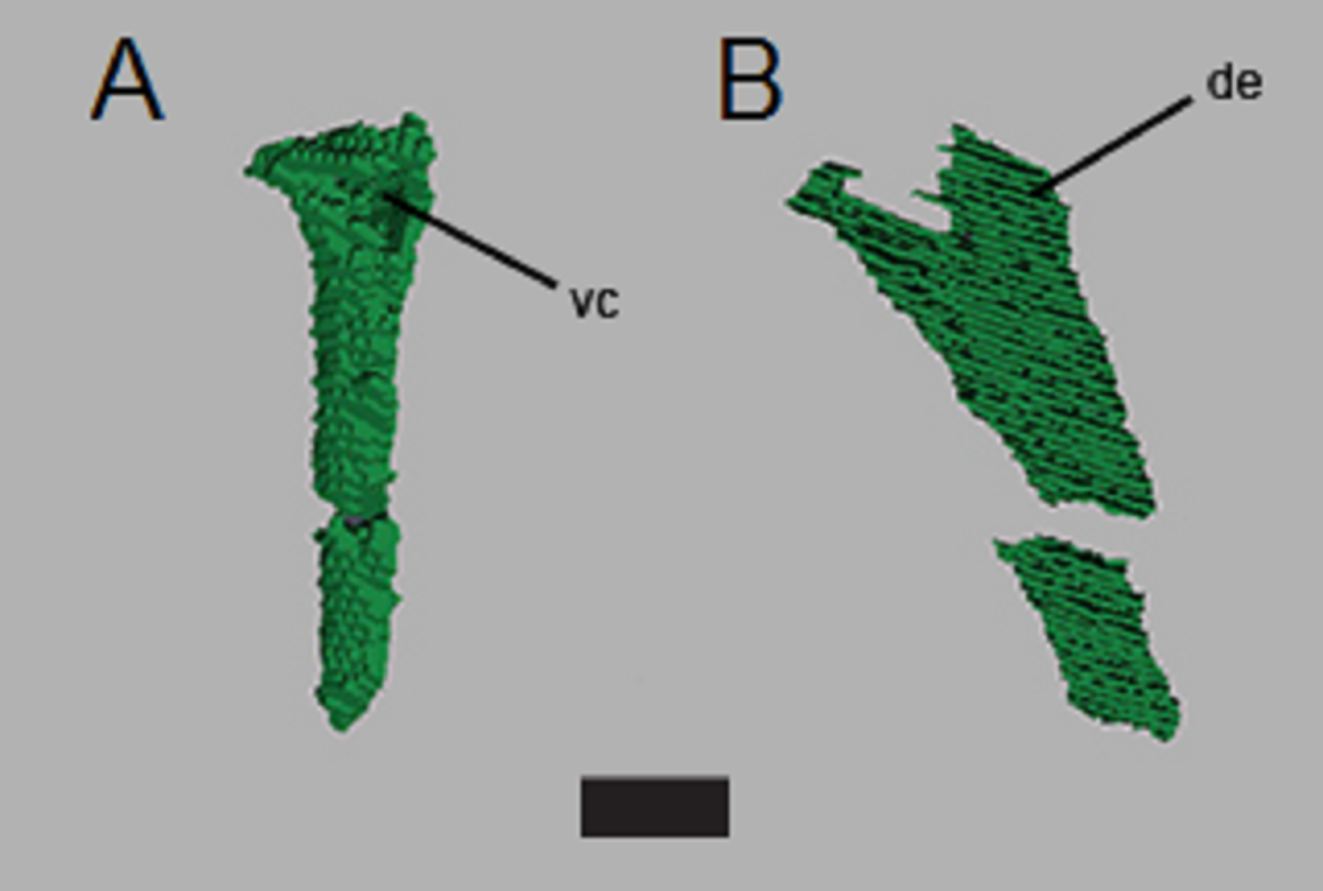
CT segmentation of left ulna. Partial ulna recovered from segmentation data in anterior view (A) and lateral view (B). Proximal end is “up” in the figure. de, dorsal expansion; vc, ventral concavity. Scale bar is 1 cm.
